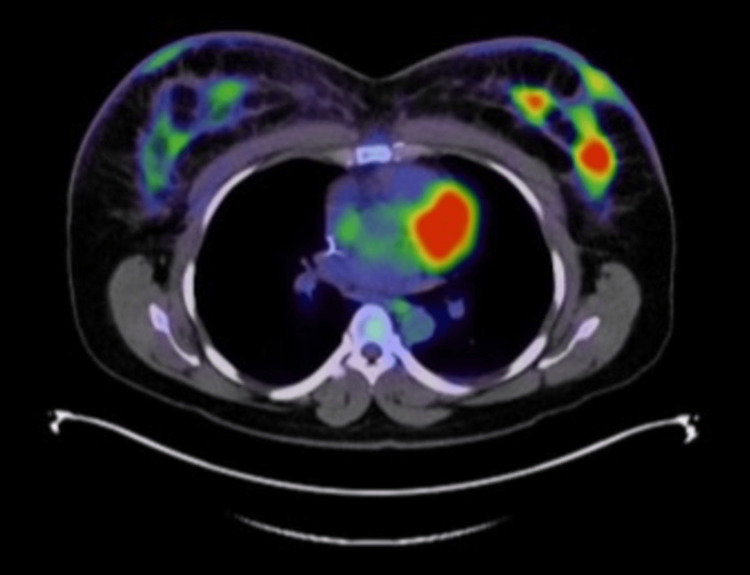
Axial PET/CT image demonstrating hypermetabolic uptake in the right and left breasts consistent with known bilateral breast cancer/malignancy. PET: positron emission tomography, CT: computed tomography
